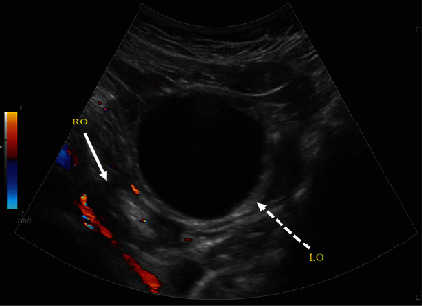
Transabdominal sagittal view of the pelvis with color Doppler, inferior to the uterus, demonstrates the right ovary (solid white arrow) with normal vascular flow. However, the left ovary (dashed white arrow), displaced by a large anechoic simple cyst, does not demonstrate vascular flow.
